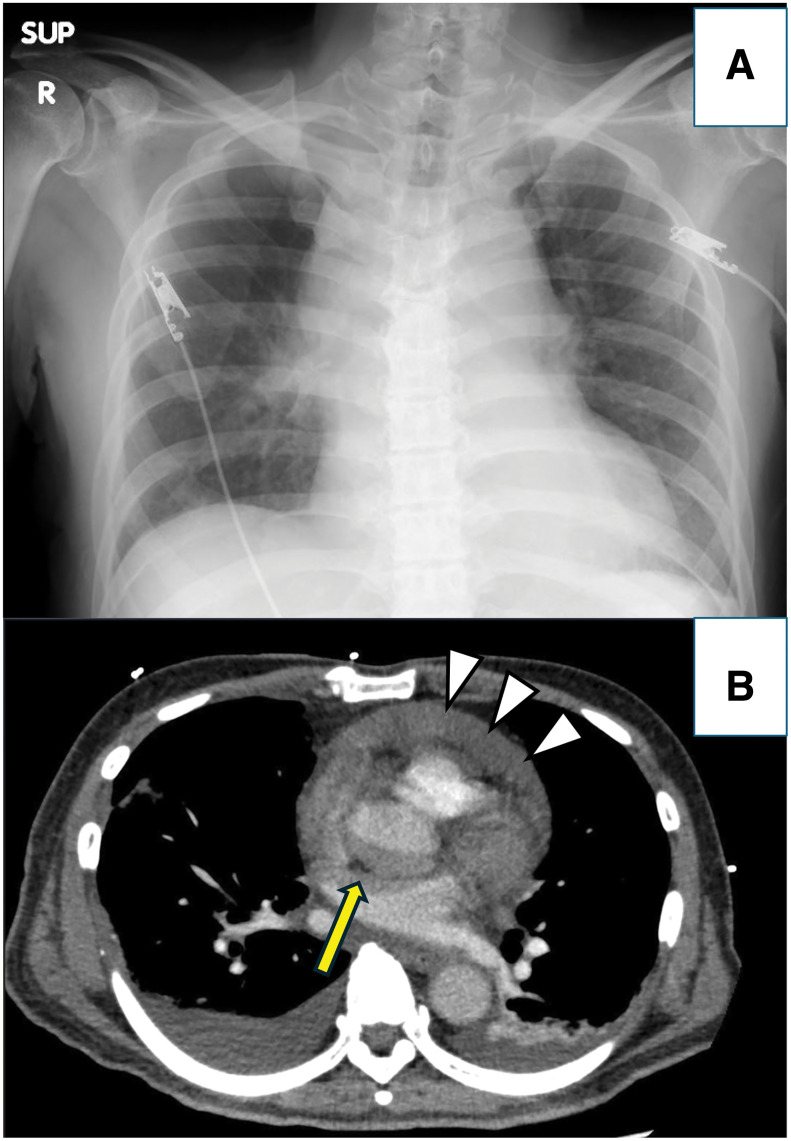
(A) A chest radiograph taken immediately after the onset of shock suggested a slight enlargement of the cardiac silhouette. The calculated CTR was 58%. (B) CT revealed evidence of pericardial effusion. Contrast density indicates blood (arrowheads). Aortic dissection was suspected based on the imaging findings (yellow arrow). CTR, cardiothoracic ratio
